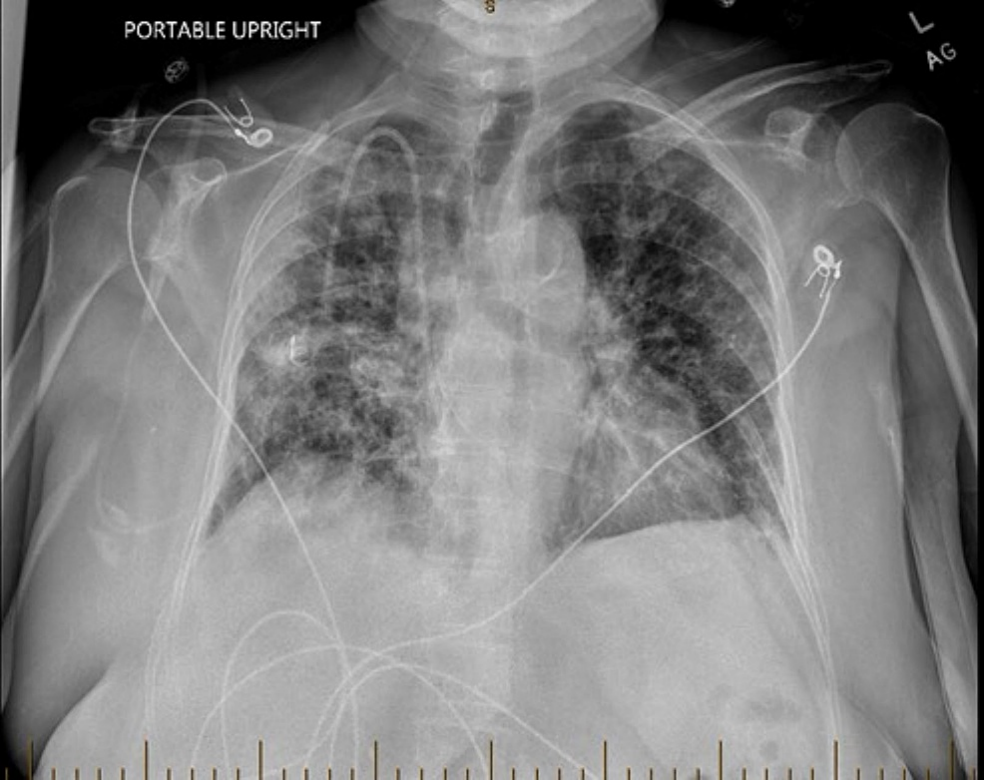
Portable chest X-ray on the day of admission showing new patchy infiltrates bilaterally.
